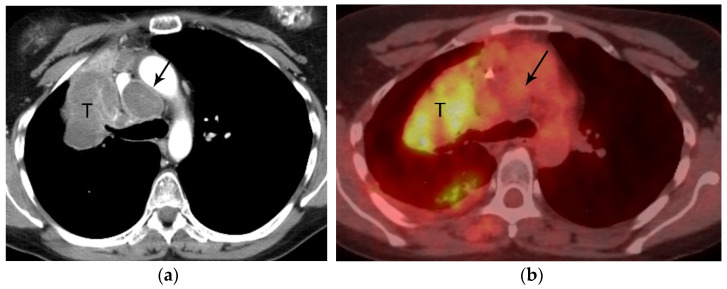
N2 nodal metastasis. (a) CT shows a 6.5 cm tumor (T) in the right upper lobe and low attenuation right paratracheal adenopathy (arrow). (b) PET/CT shows FDG avidity of the primary tumor (T) but the biopsy-proven N2 ipsilateral nodal metastasis is not FDG avid. Necrotic nodal metastases can give false negative results on PET/CT.
